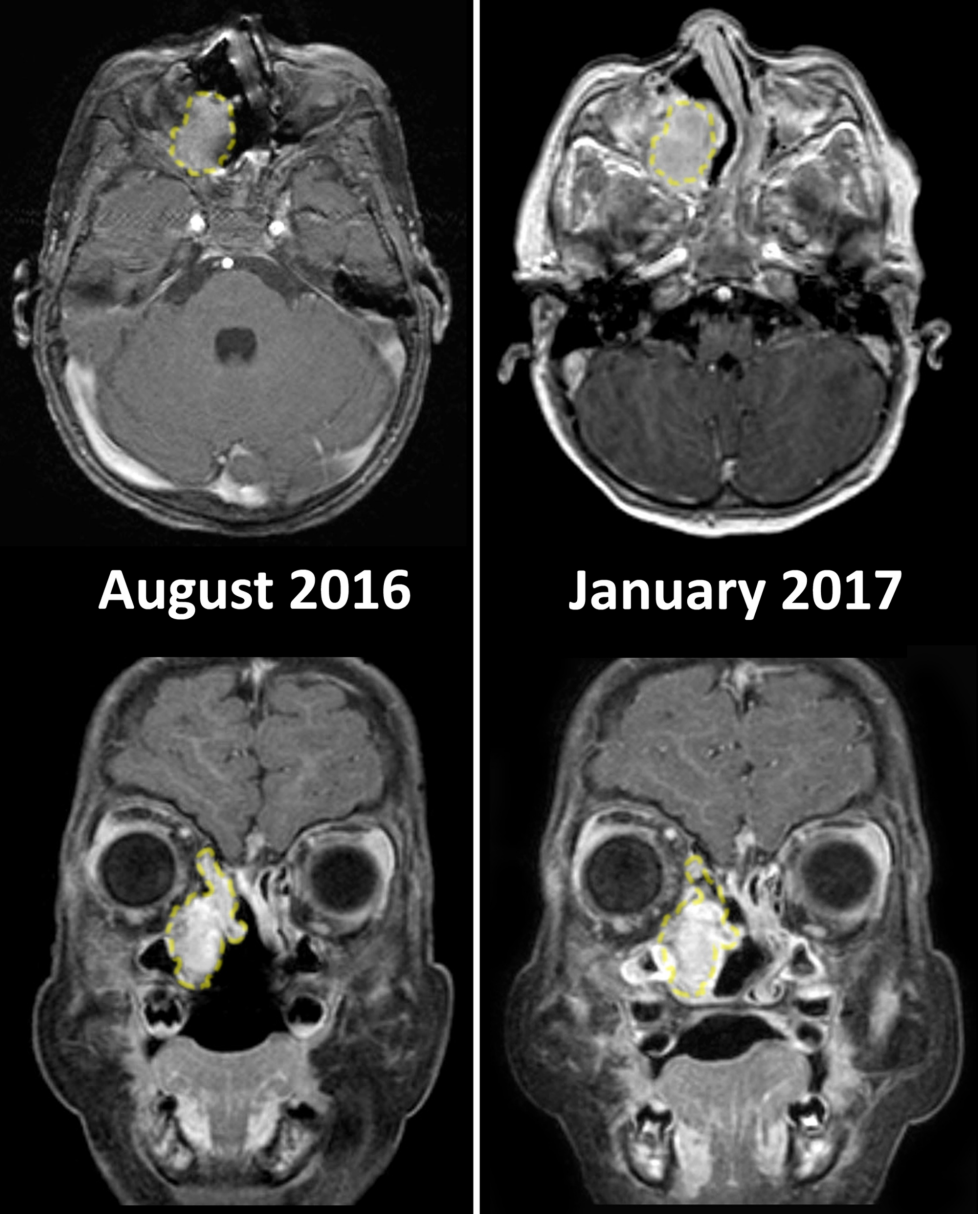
Follow-up MRI after the first surgery In August 2016, (left) images revealed recurrence of the tumor eight months after surgery, however, without significant mass effect. Comparison of the corresponding scans between August 2016 and January 2017 (right) suggests the increase in dimensions of the lesion, which meant that it was not stable. To demonstrate the difference in size, the outline of the tumor on the earlier scans is positioned over the later images (dashed line).
